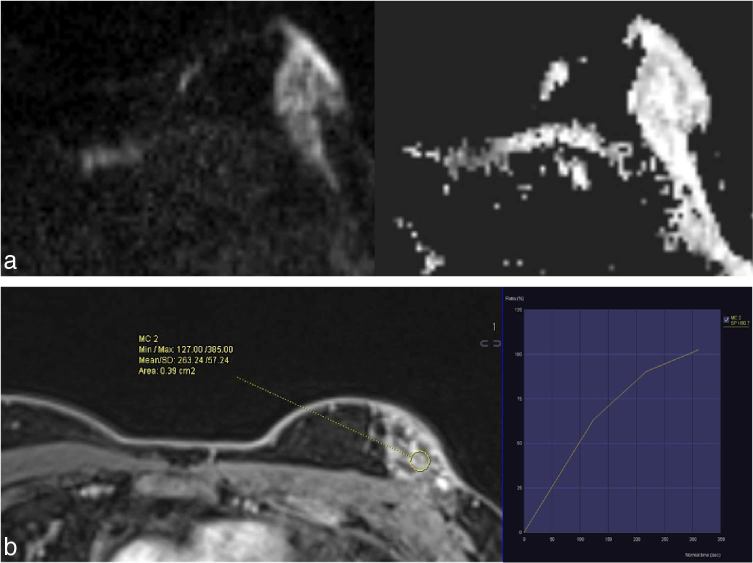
MRI of the left breast shows no evidence of restriction on diffusion-weighted imaging (a) and Type I kinetic enhancement curve (b).
